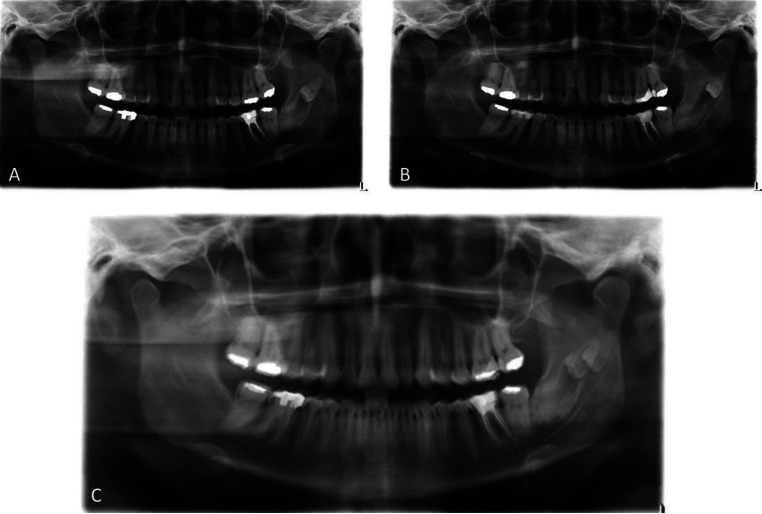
Example of two panoramic radiographs performed in 2009 (A) and in 2014 (B), and an example of the superposition (C) performed to analyse the migration over time of the third molar.
